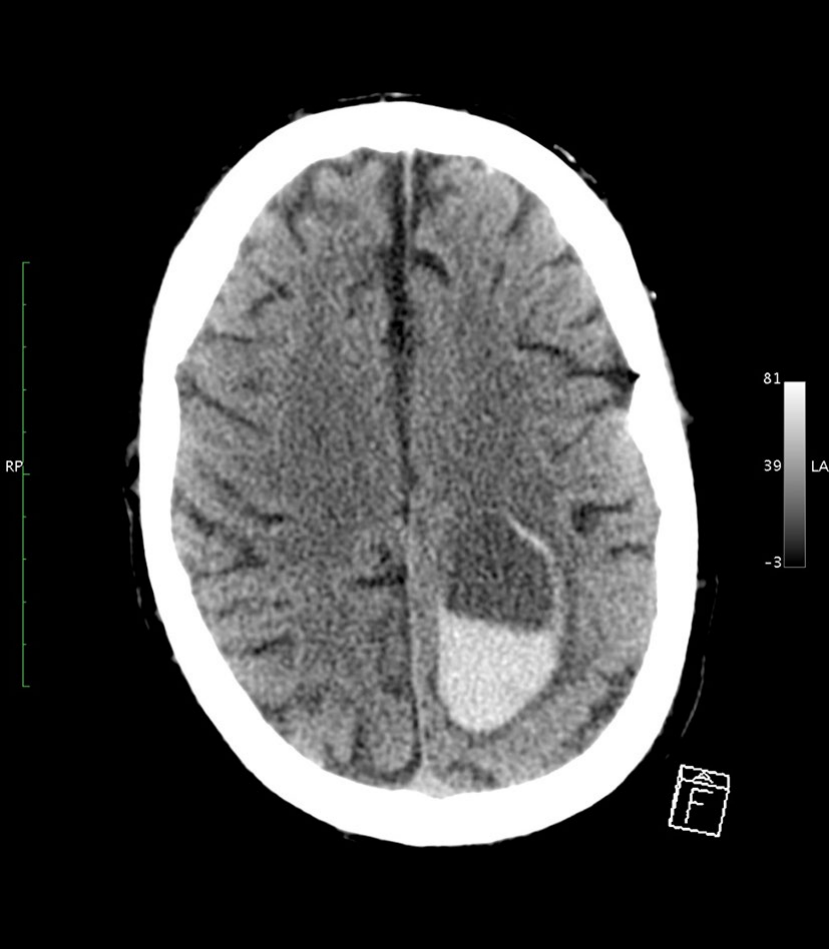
Typical blood-fluid level on an acute CT scan of a patient with an intracerebral hemorrhage in the left parietal lobe.
